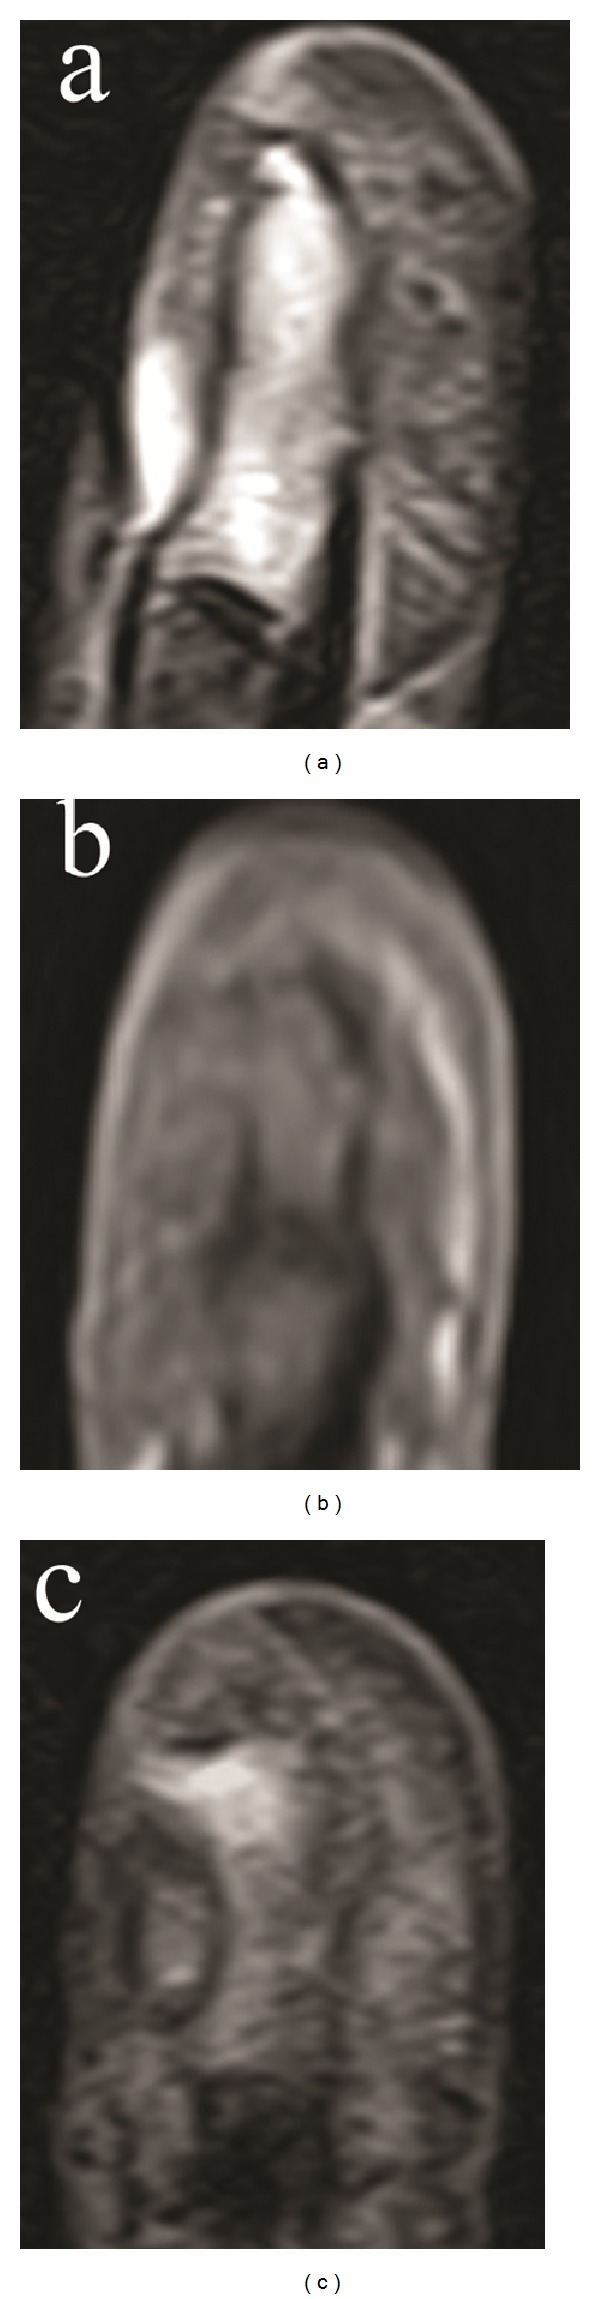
Sagittal view of short time inversion recovery magnetic resonance images (MRI) (a) and coronal views of T1 weighted (b) and T2 weighted (c) MRI.
